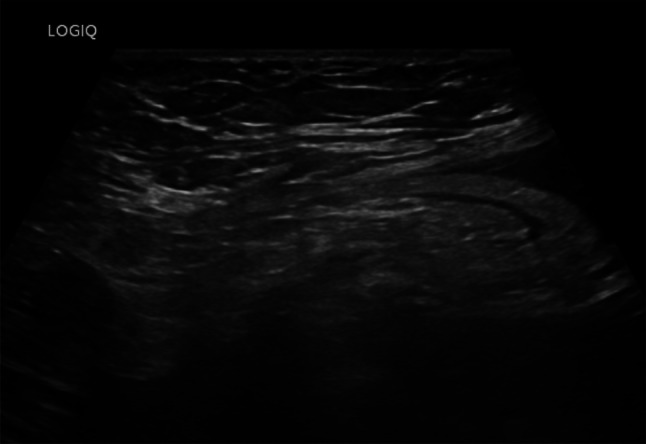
In the left inguinal region, a hernia orifice measuring 10 mm is visible, through which omental fat is protruding, showing weak arterial signals on Doppler imaging. Additionally, a small amount of peri-omental fluid is present
